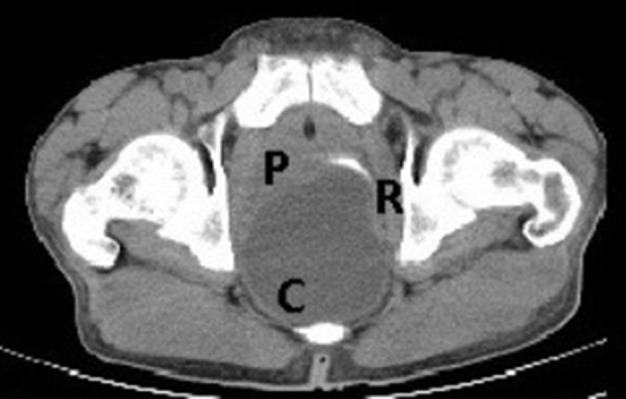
CT NC axial: a well-defined thin-walled smooth cystic SOL (C) compressing the anteriorly displaced rectum (R). UB, urinary bladder.
